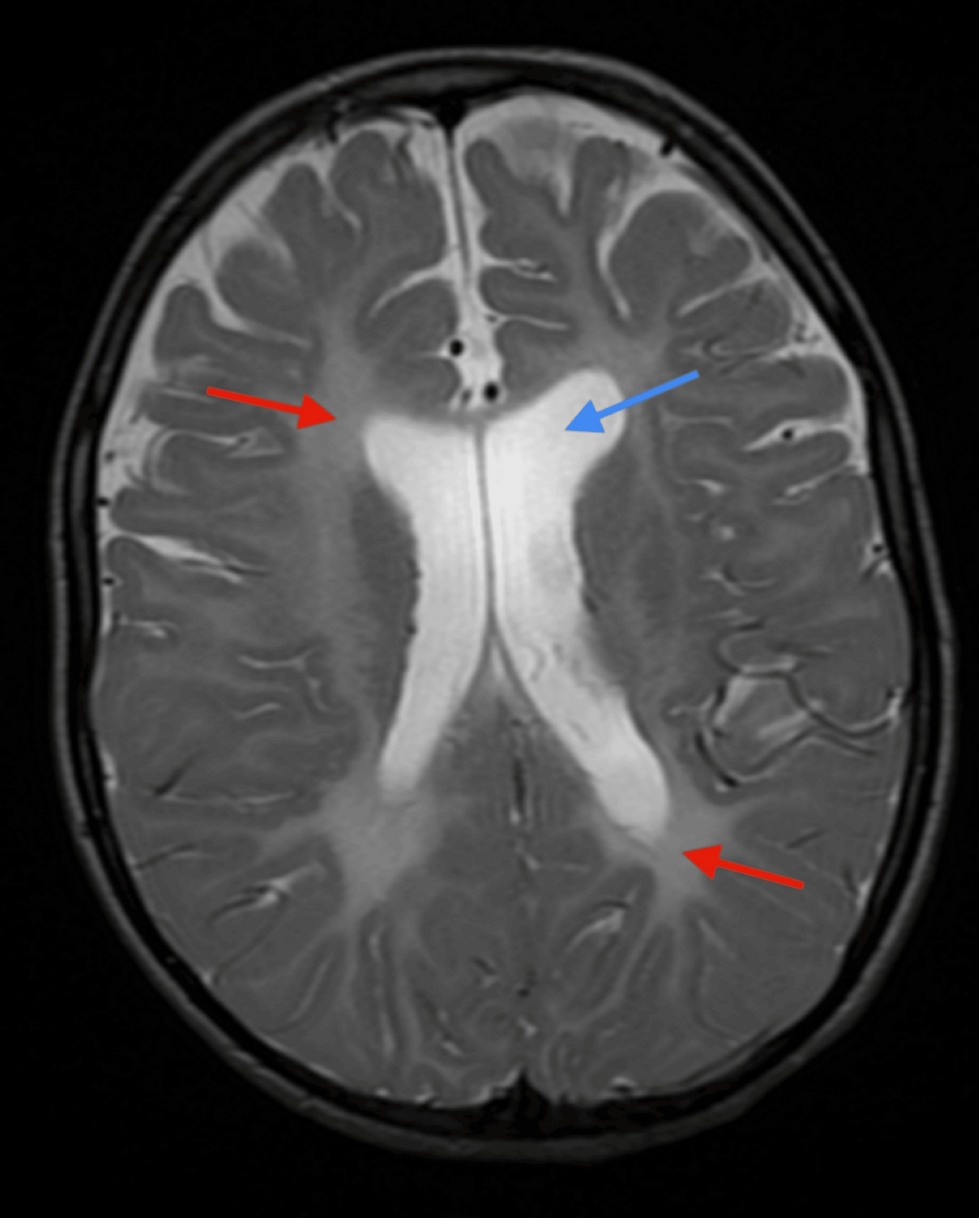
Axial T2-weighted imaging at the level of the basal ganglia The basal ganglia are normal in this case. Prominent perivascular spaces were noted. There is diffuse bilateral white matter T2 hyperintensity (red arrows) and relative cerebral volume loss of the frontal lobes with dilatation of the lateral and third ventricles (blue arrow).
